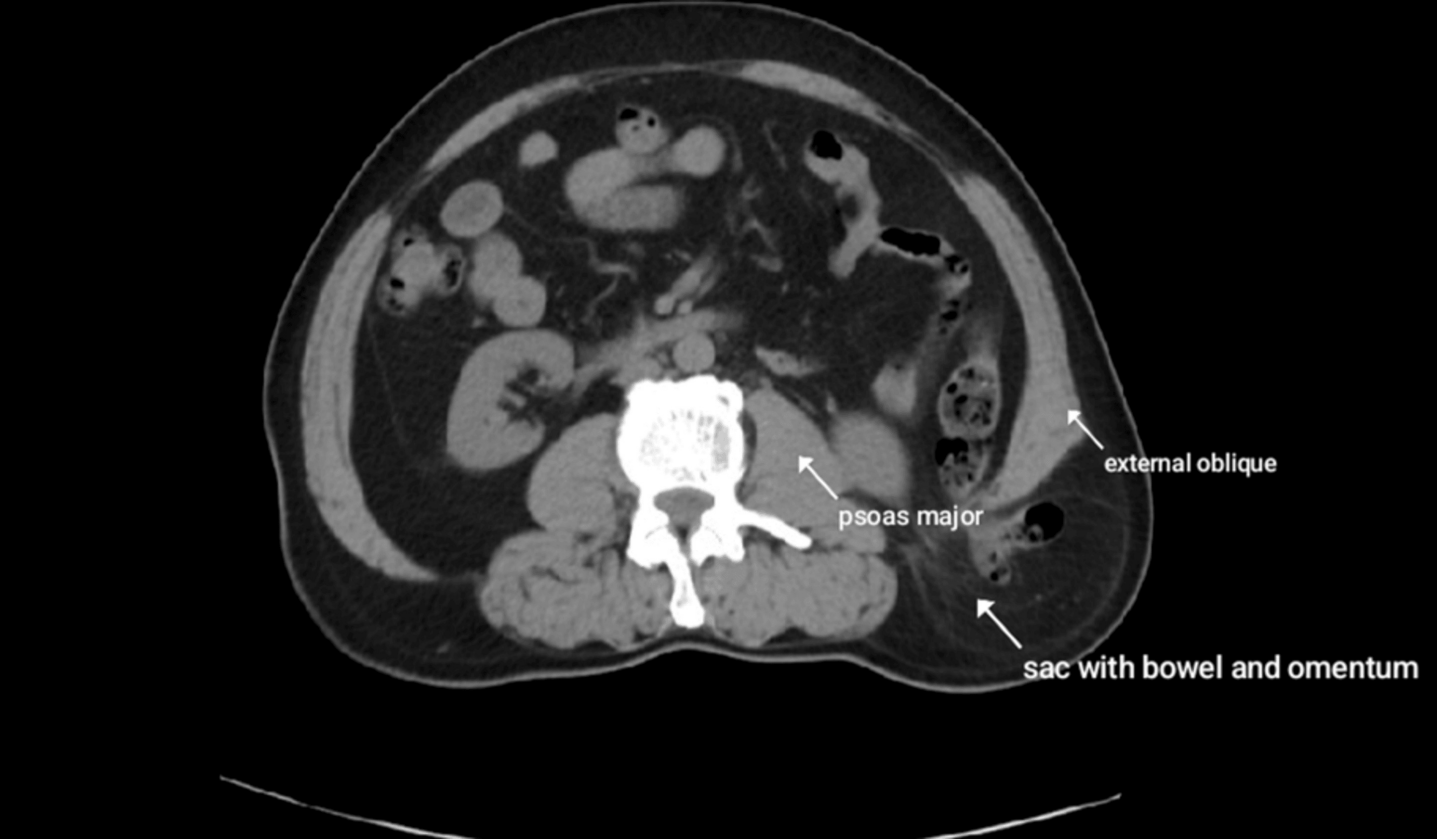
CT scan demonstrating an inferior lumbar hernia with omentum and bowel as content.
